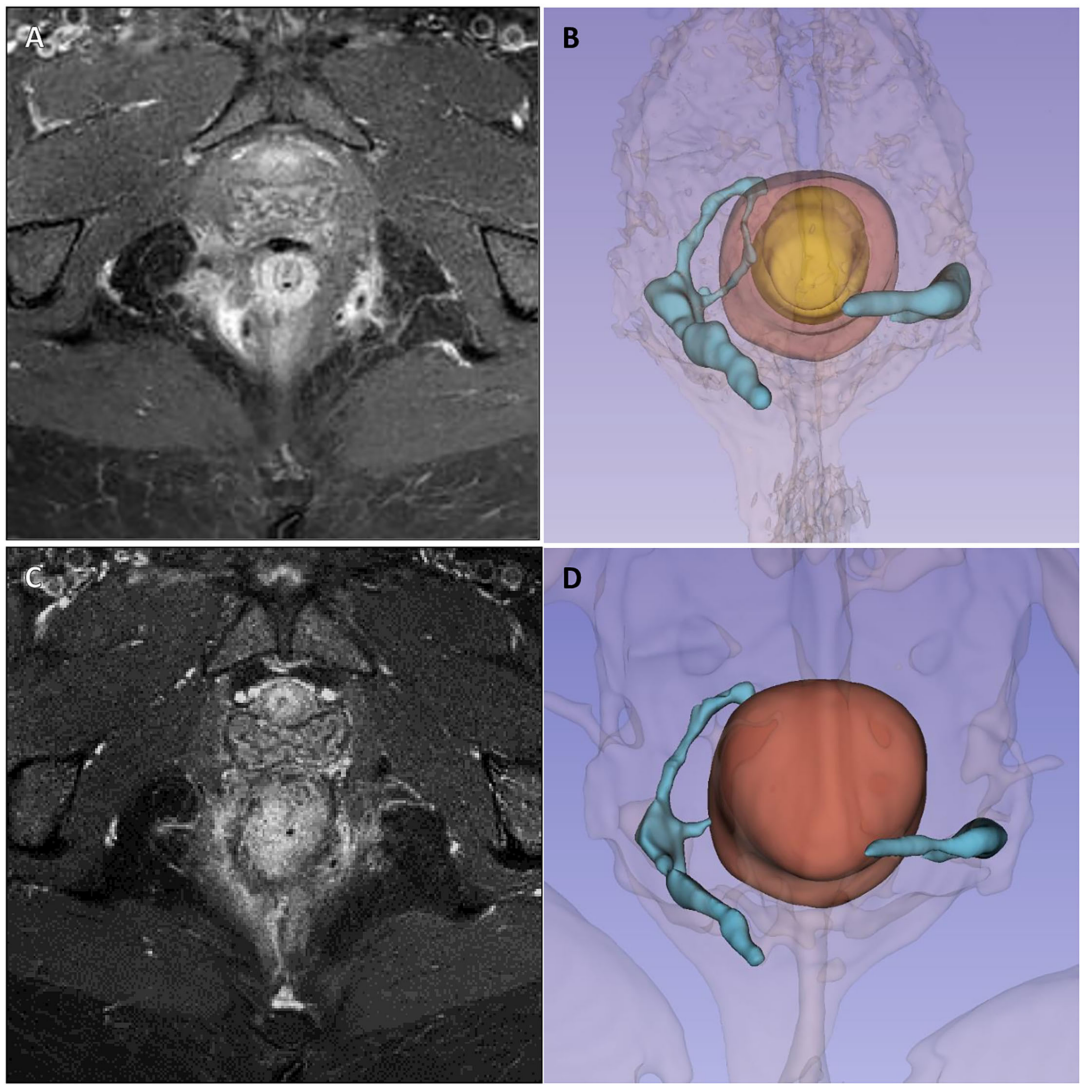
Case 2 imaging pre- and post-surgery. Panels (A, B) show pre-operative pelvic magnetic resonance imaging and 3D reconstruction showing two active complex trans-sphincteric fistulas: on the left a trans-sphincteric fistula with a seton in place, and on the right a branched trans-sphincteric fistula with an abscessual cavity of 17 × 10 mm in the ischioanal space and a single external orifice in the posterior right perianal quadrant (h8). Panels (C, D) show 6-month MRI follow-up, with persistence of fistula tracts but with less thickness and no related collections.
